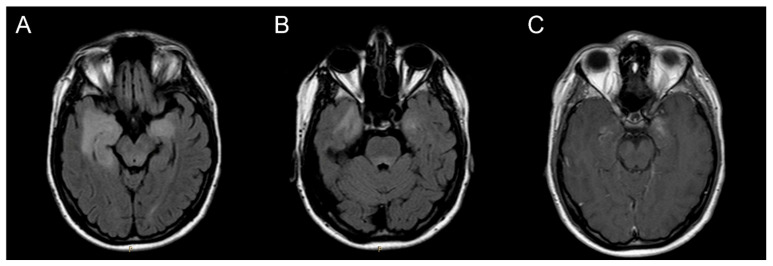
FLAIR bilateral mesial temporal hyperintensity involving the mesial temporal lobes (A) and the periventricular and dorsal area of the pons (B). Gadolinium-enhanced MRI sequence showing bilateral enhancement (C).
